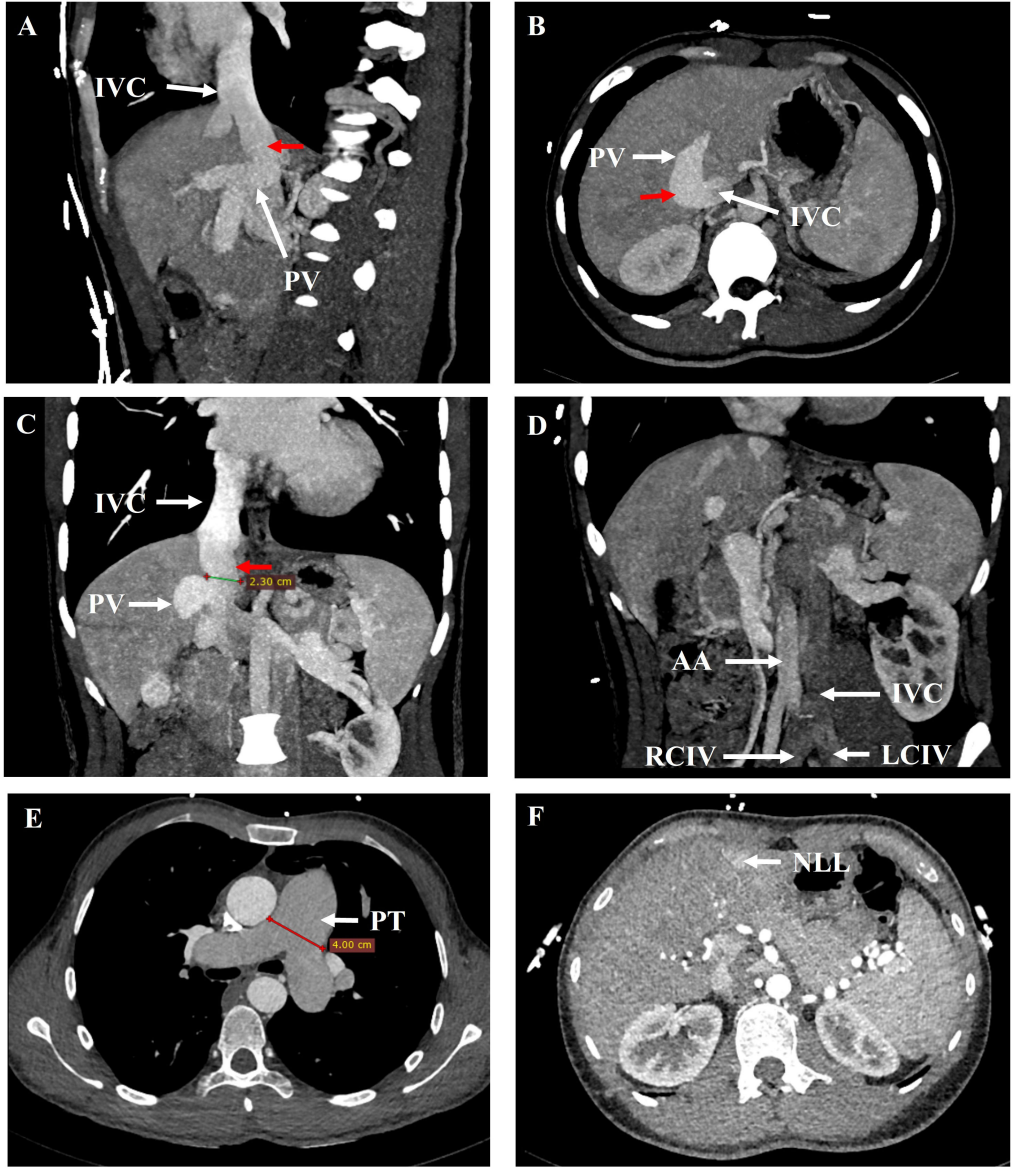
21 years-old male with Abernethy malformation type II, left ICV, and NLL. (A) Sagittal, (B) axial and (C) coronal maximum intensity projection image from contrast-enhanced CT revealed a vessel communication (red arrow) between the PV and the IVC. (D) Oblique coronal maximum intensity projection image demonstrated the IVC was on the left side of the abdominal aorta. (E, F) Arterial phase axial images from contrast-enhanced CT showed the dilated pulmonary trunk and the nodular liver lesions (NLL). AA, abdominal aorta; IVC, inferior vena cava; LCIV, left common iliac vein; NLL, nodular liver lesions; PV, portal vein; PT, pulmonary trunk; RCIV, right common iliac vein.
